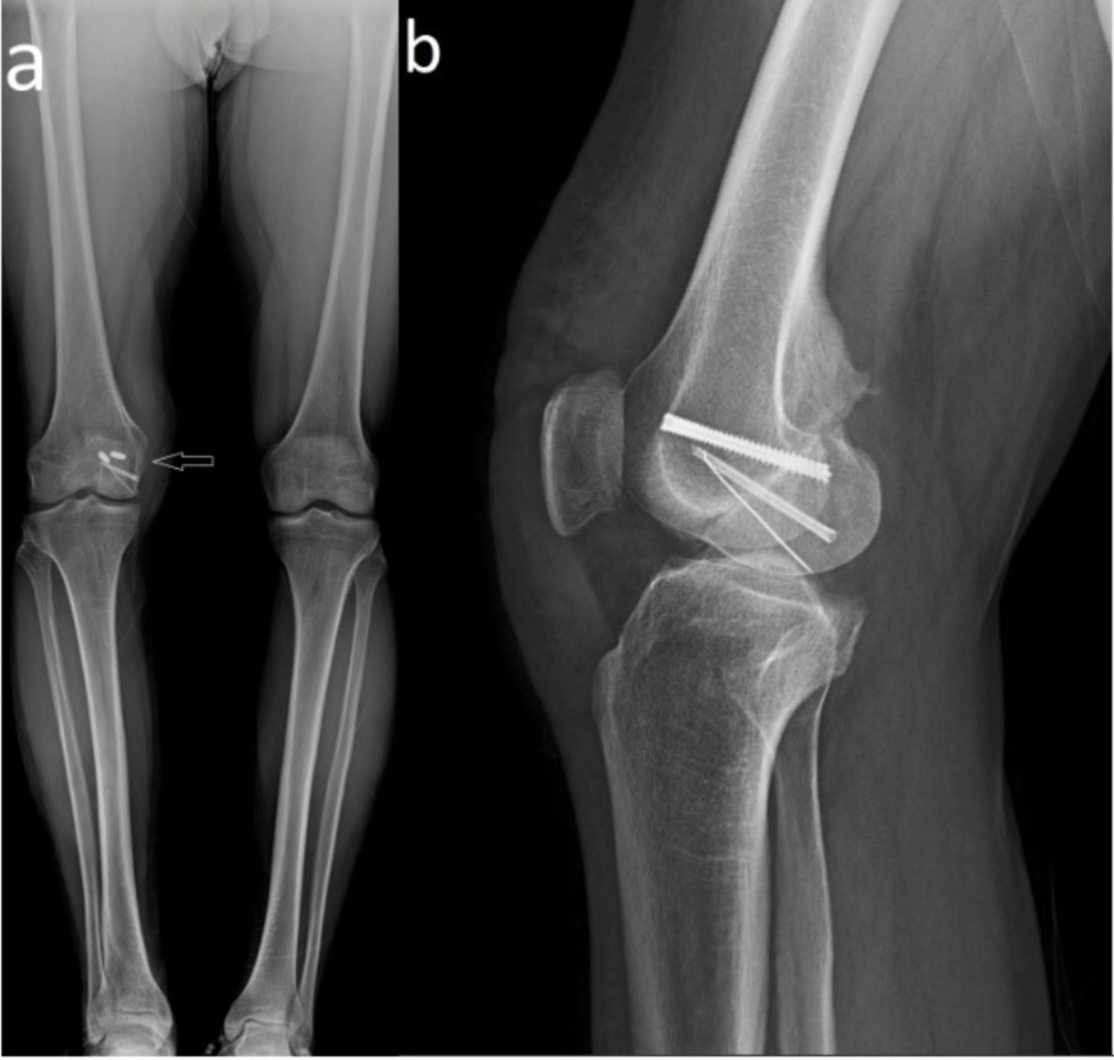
Radiographic images at the end of the follow-up (a) Anteroposterior orthoroentgenogram (arrow) and (b) lateral radiographic images at the end of the follow-up of the patient.
